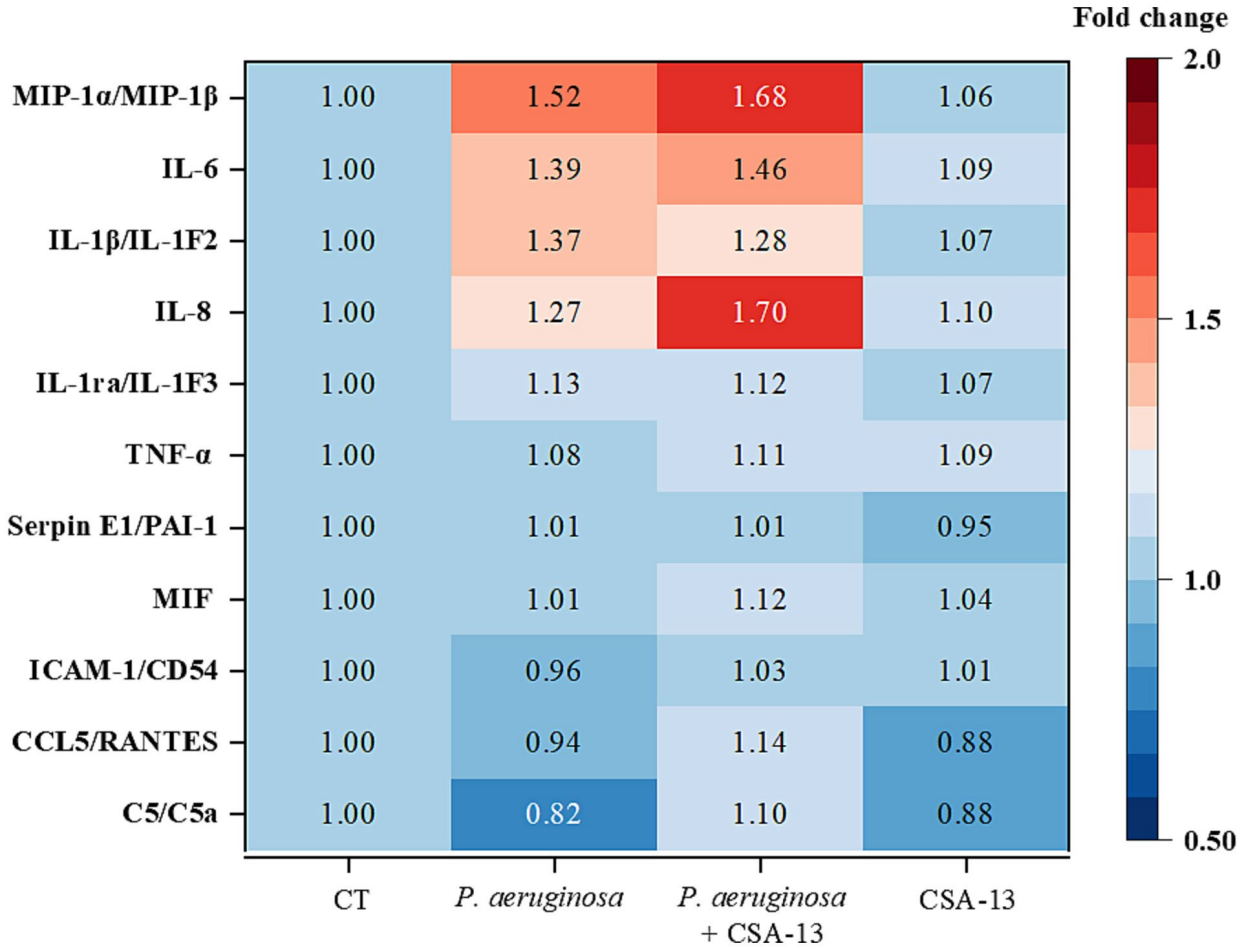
Determination of cytokine levels in plasma after the addition of CSA-13 at a dose of 20 μg/mL (data expressed as fold change compared to the untreated condition, CT). Warmer colors suggest increased cytokine expression, while colder colors suggest decreased expression.
